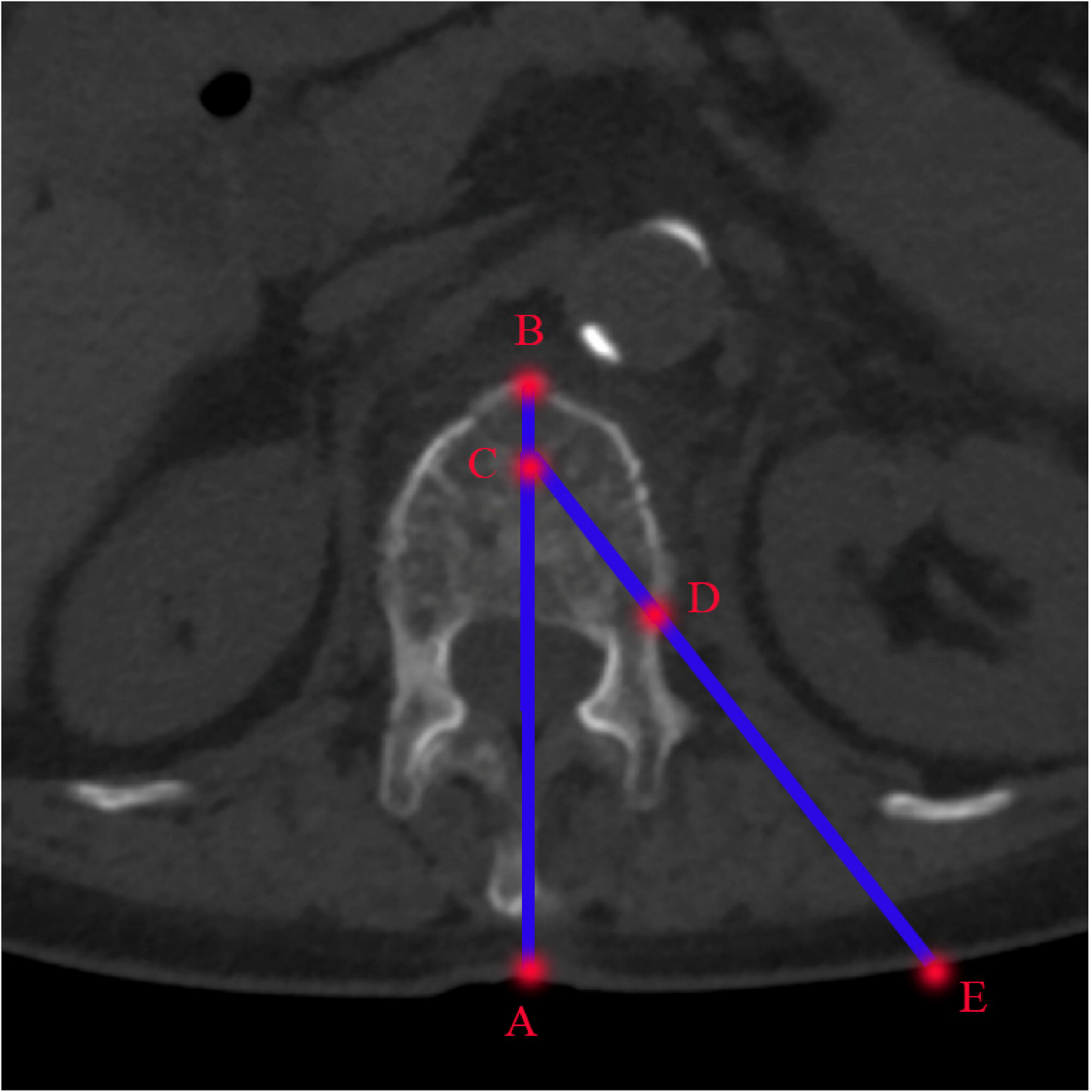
The measurement of puncture angle and distance based on preoperative cross-sectional CT scan. Line AB: middle line of the fractured vertebra; Point C: the anterior one-third point of the vertebral body on the middle line; Point D: the superolateral junction between the pedicle and the fractured vertebra. Point E: the intersection of line CD and skin surface. Line AE is the distance between the skin entry point and spinous process and Angle between lines AB and CE is the puncture angle.
